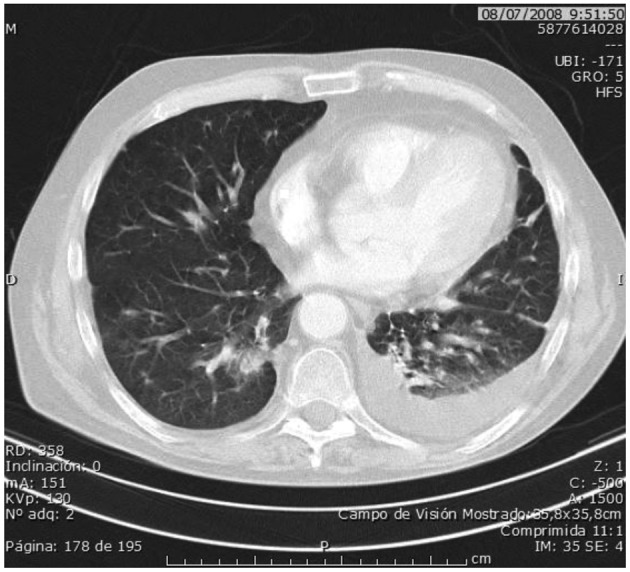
July 2008: The CT scan revealed new pulmonary nodules and pleural effusion.
